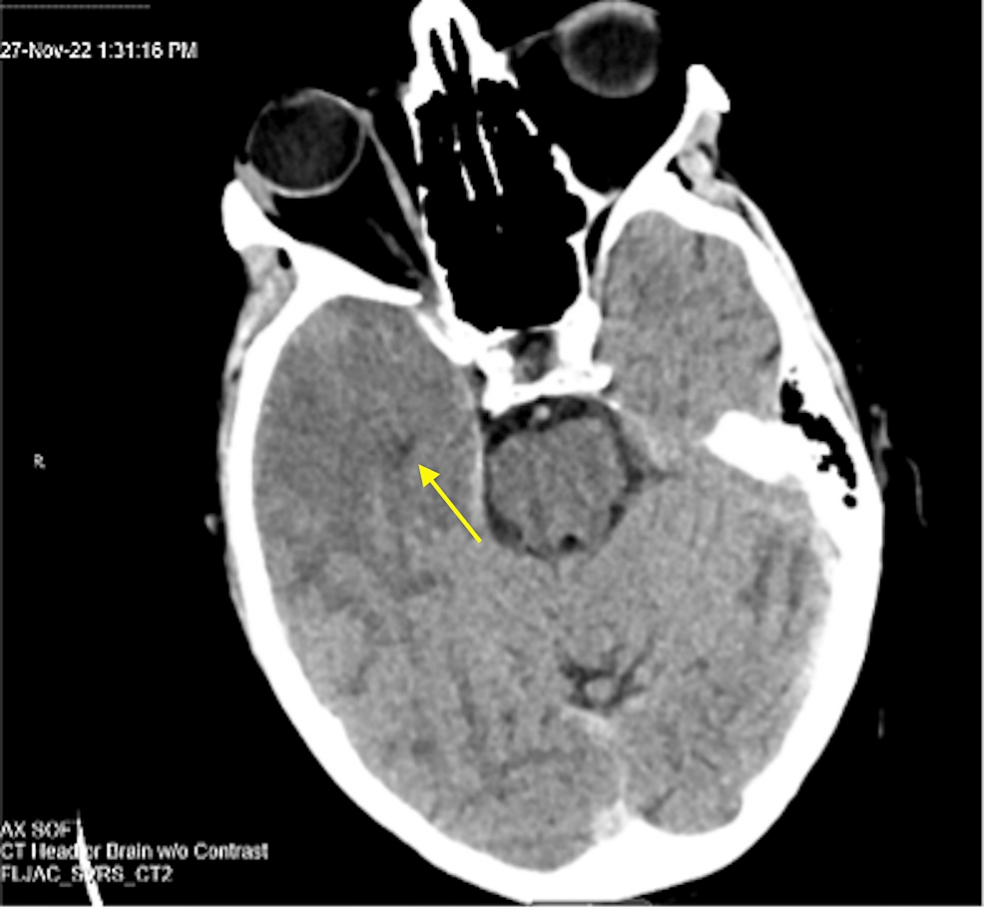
CT head or brain without contrast revealed a zone of hypoattenuation within the region of the right temporal lobe.
